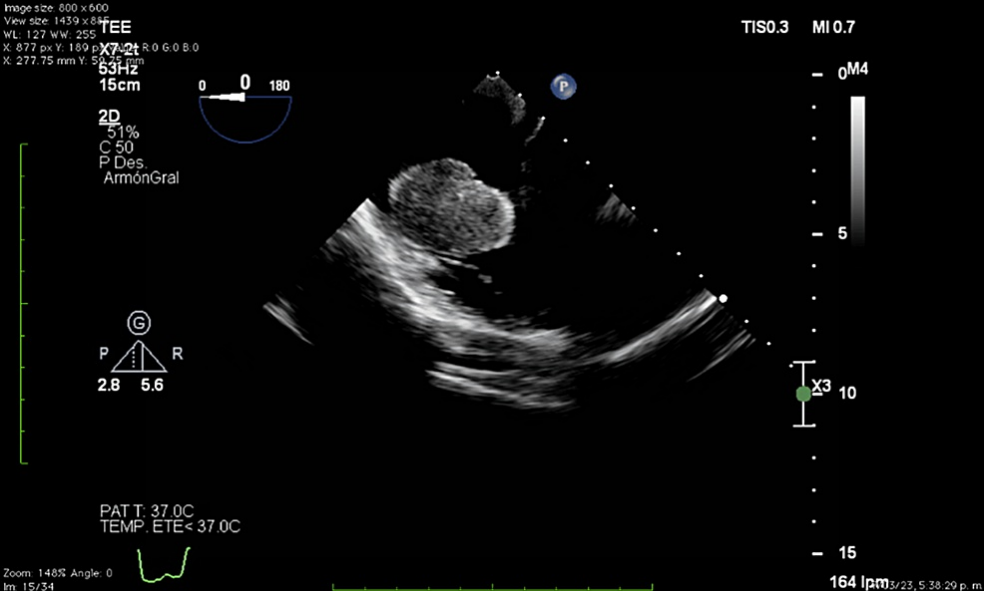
Transthoracic echocardiogram revealed a solid mass measuring 42 mm x 41 mm originating from the lateral wall of the right atrium
